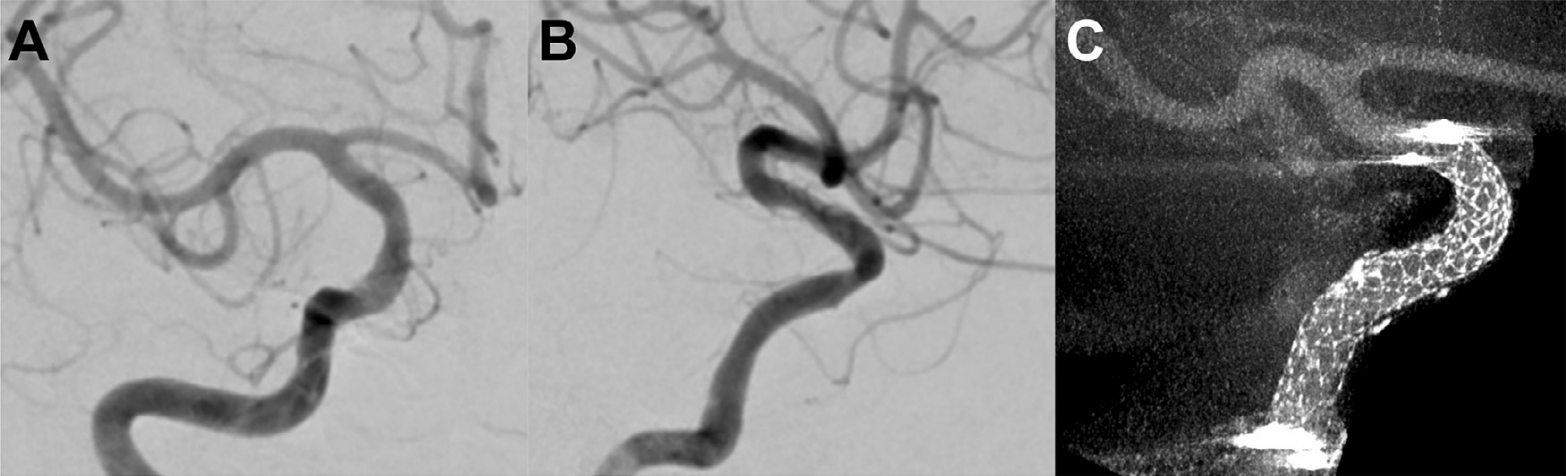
Follow-up imaging. (A and B) Six months after the procedure, follow-up angiography in the frontal and lateral projections showing resolution of dissection with minimal stenosis. (C) Cone-beam computed tomography imaging showing sufficient stent-wall apposition.
